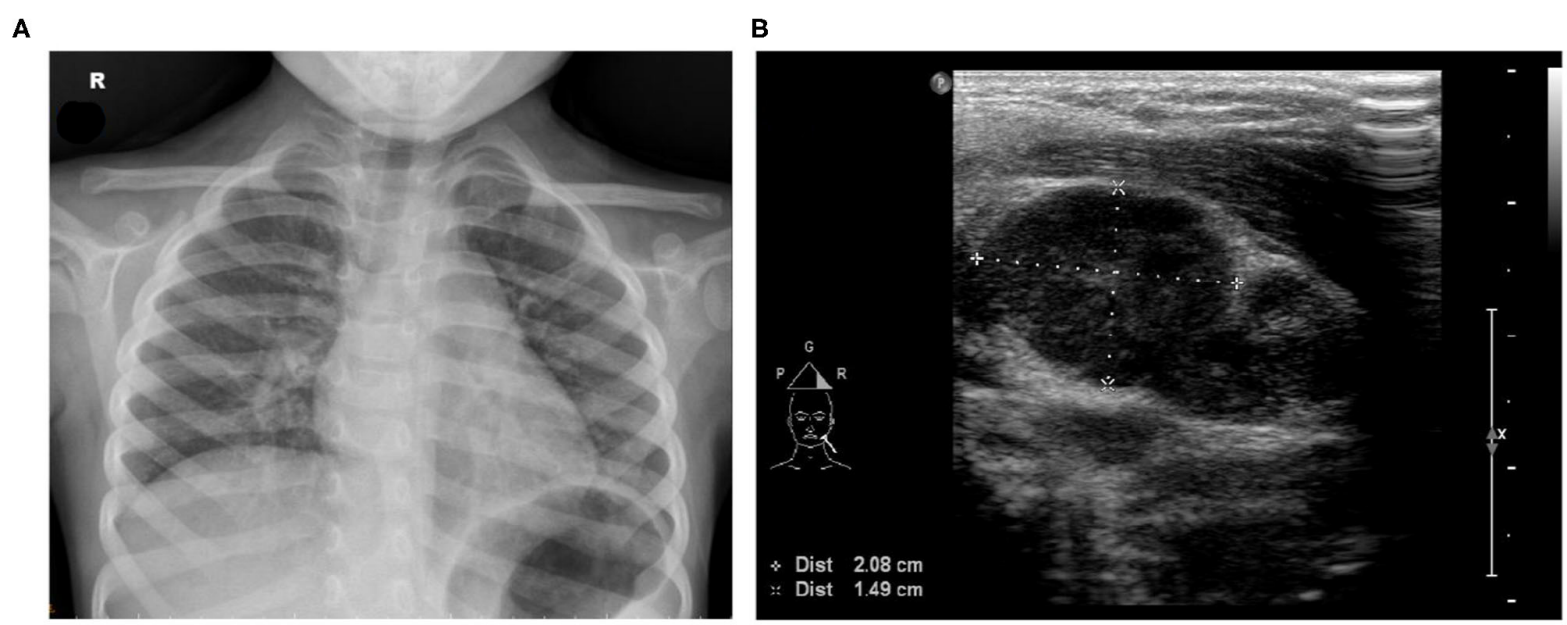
(A) Chest X-ray image exhibiting increased bronchoalveolar infiltration over the right lung field. (B) Neck tissue echography revealing multiple lymphadenopathy on the left side of the neck (largest dimensions: 2.08 × 1.49 cm).
